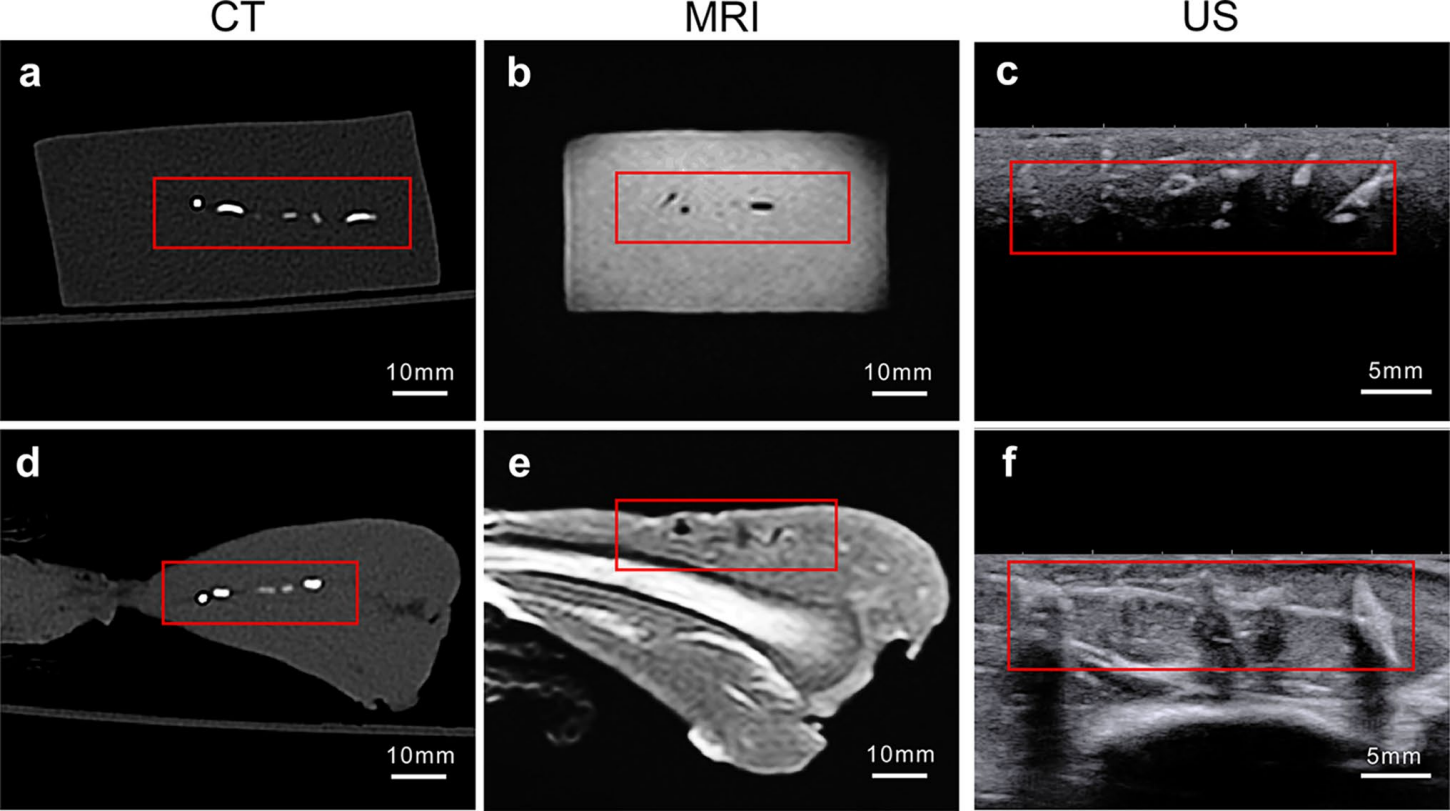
Results of common imaging diagnostic methods. a, d CT scan results of metal, glass, wood, plastic, graphite, and ceramic foreign bodies in adipose and muscle tissues. b, e MRI scan results of glass, wood, plastic, and ceramic foreign bodies in adipose and muscle tissues. c, f Ultrasound imaging results of metal, glass, wood, plastic, graphite, and ceramic foreign bodies in adipose and muscle tissues. The red box indicates the location of the foreign bodies
